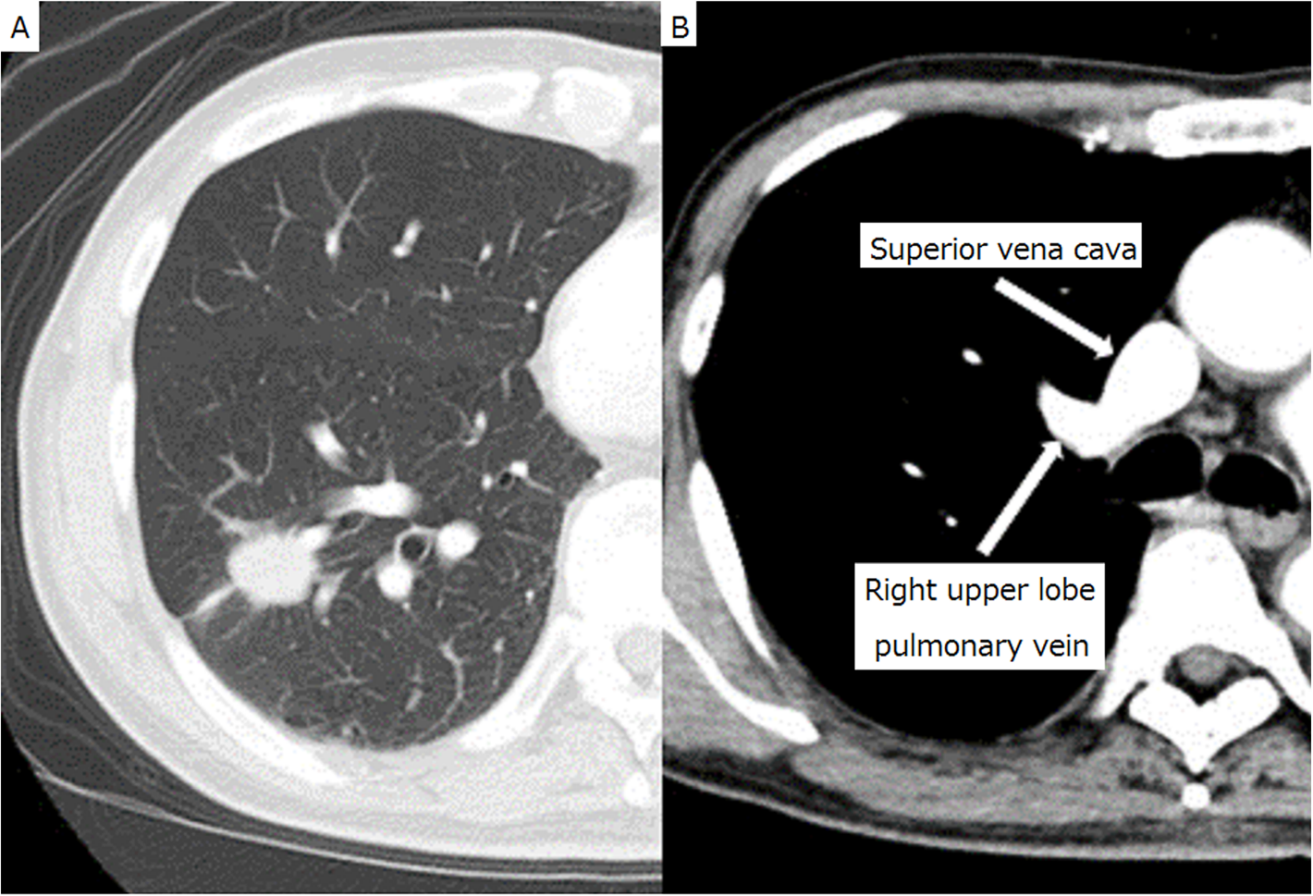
Computed tomography. Thoracic computed tomography showed a tumor shadow in the right lower lobe (a) and an enhanced anomalous vein (b)
